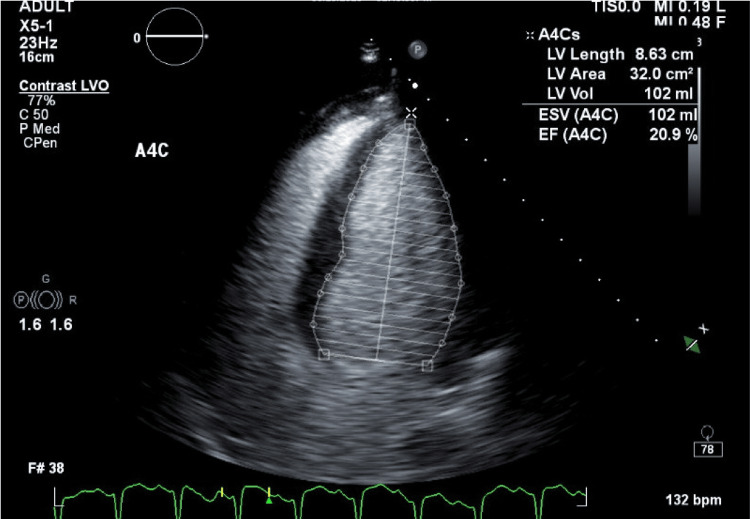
Echocardiogram at presentation showing left ventricular ejection fraction of 20%.
